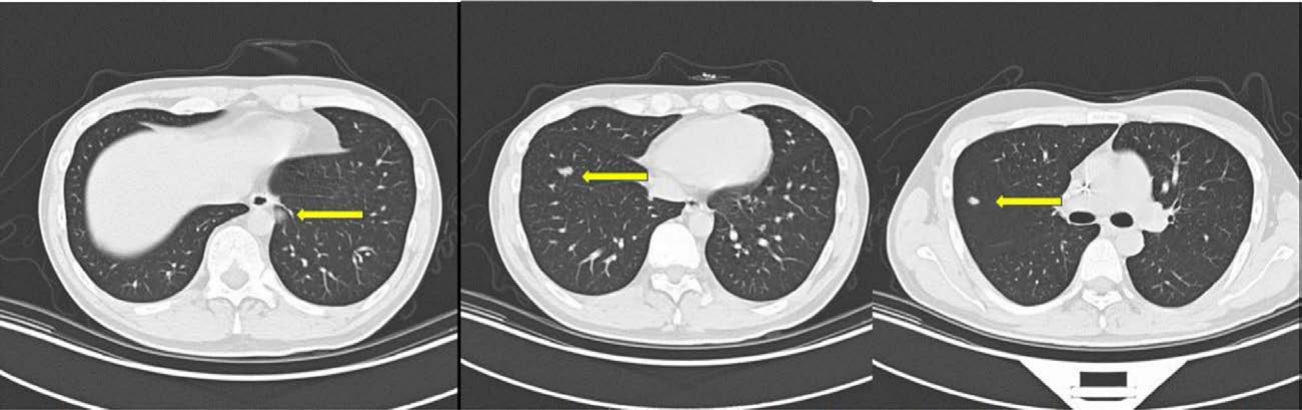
Chest CT of Case 4. CT = computed tomography.
